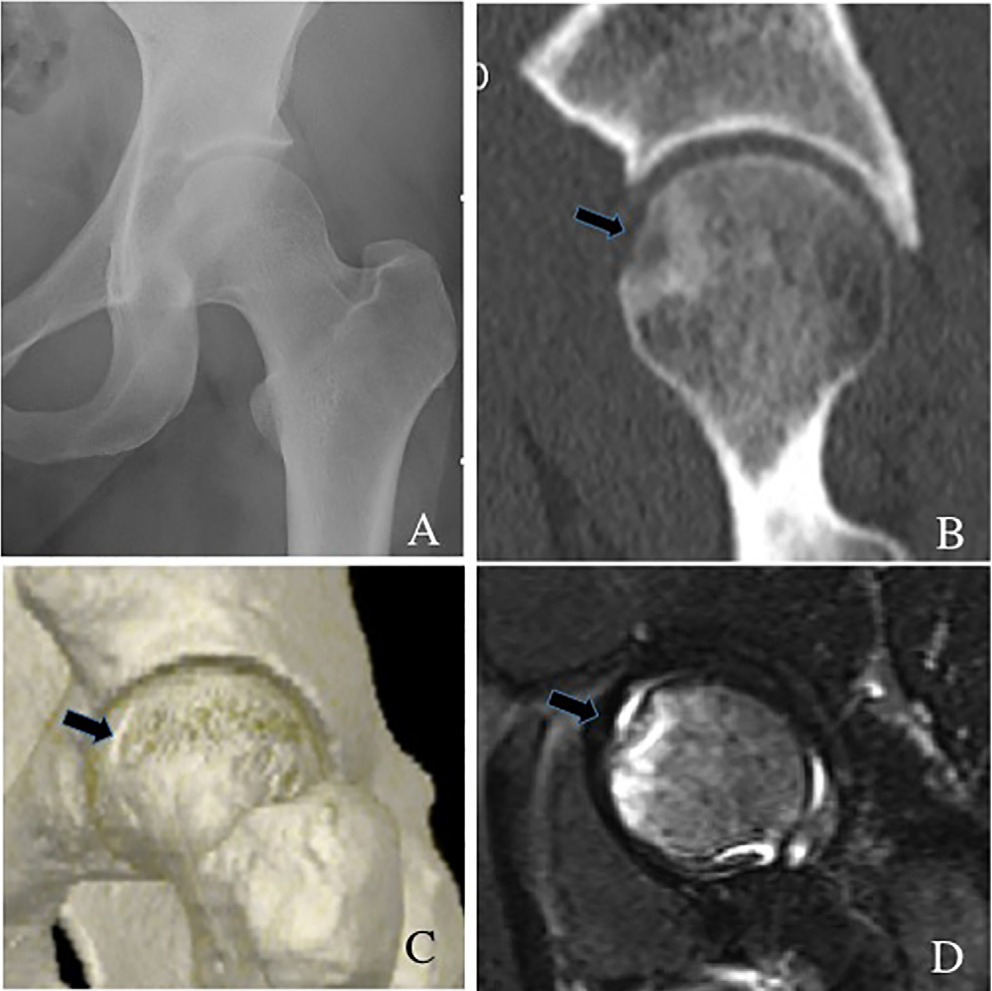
(A) A round radiolucent lesion (arrow) suggests an osteochondritis dissecans (OCD) lesion at the anterosuperior portion of the left femoral head. (B) Preoperative oblique sagittal view of computed tomography showing a round radiolucent lesion at the anterosuperior site of the femoral head. (C) 3-dimensional computed tomography also showing an OCD lesion (arrow) at the anterosuperior site of the femoral head. (D) Coronal magnetic resonance imaging (MRI) view (short inversion time inversion-recovery sequence) showing a high-intensity round lesion (arrow) at the anterosuperior portion of the femoral head.
